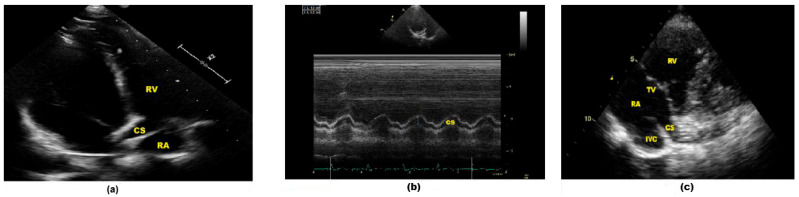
Transthoracic echocardiography-based evaluation of CS flow parameters. (a) Modified apical four-chamber imaging allows for clear visualization of the CS opening into the right atrium; (b) systolic and diastolic CS diameters were obtained via M-mode in the modified apical four-chamber view; (c) entry of the CS into the RA was identified using the modified parasternal long-axis view. Abbreviations: coronary sinus (CS), inferior vena cava (IVC), right atrium (RA), right ventricle (RV), tricuspid valve (TV).
